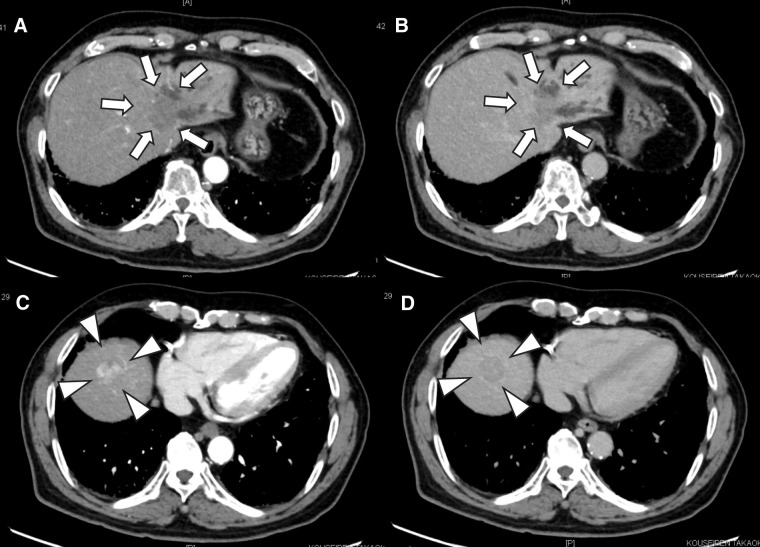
Contrast enhanced dynamic CT images revealing peripheral enhancement with central low density in the arterial phase (A) and increased enhancement in the portal venous phase (B) at segments IV, II, and III (white arrows). In addition, the tumor at segment VIII is heterogeneously enhanced in the arterial phase (C), followed by wash-out in the portal venous phase (D) (white arrowheads).
